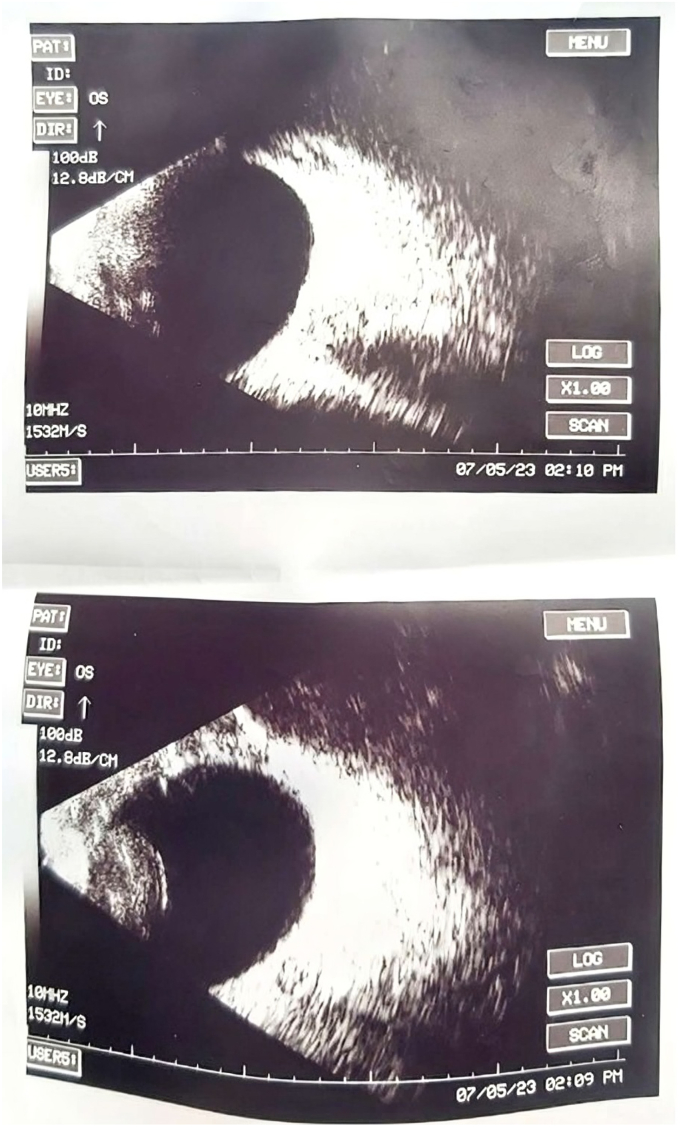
Ocular ultrasound - edematous lenses and minimal debris in the vitreous humor.
